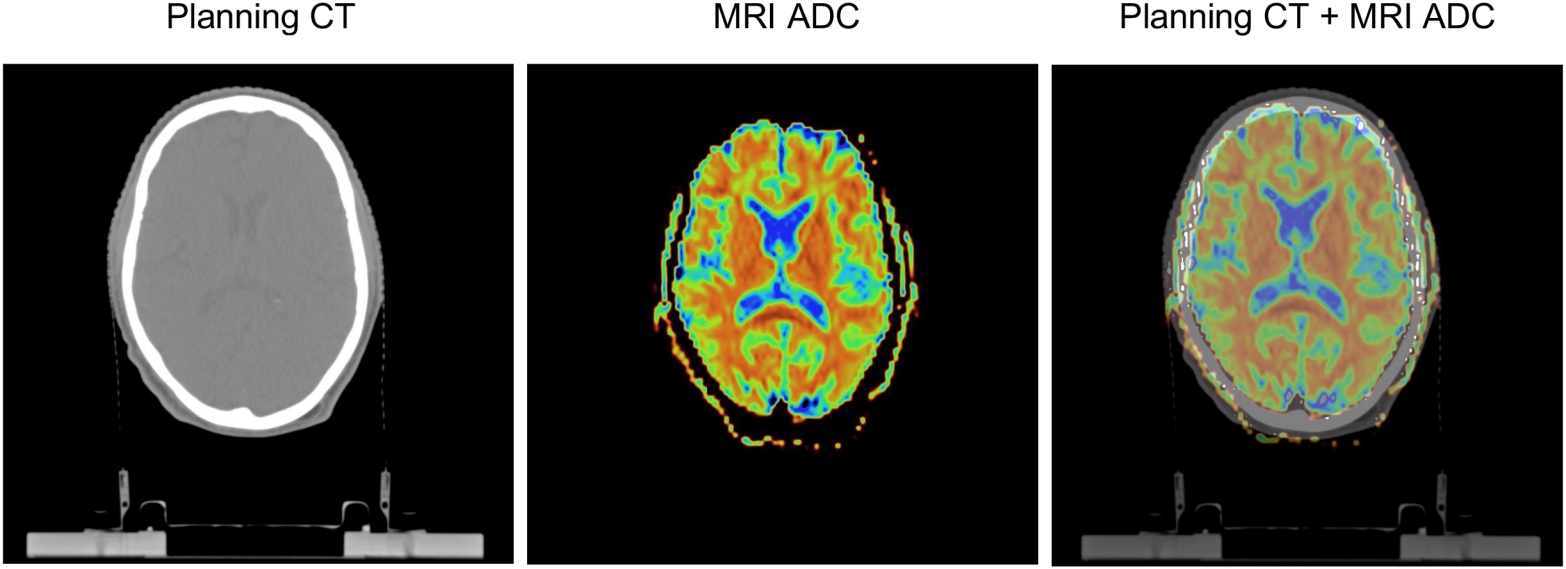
Rigid co-registration of MRI apparent diffusion coefficient (ADC) maps and planning CT. Images shown are obtained in a patient aged 51 years at RT onset, diagnosed with an oligodendroglioma in the right frontal lobe (not shown), and 46 months between the end of RT and the MRI scan.
